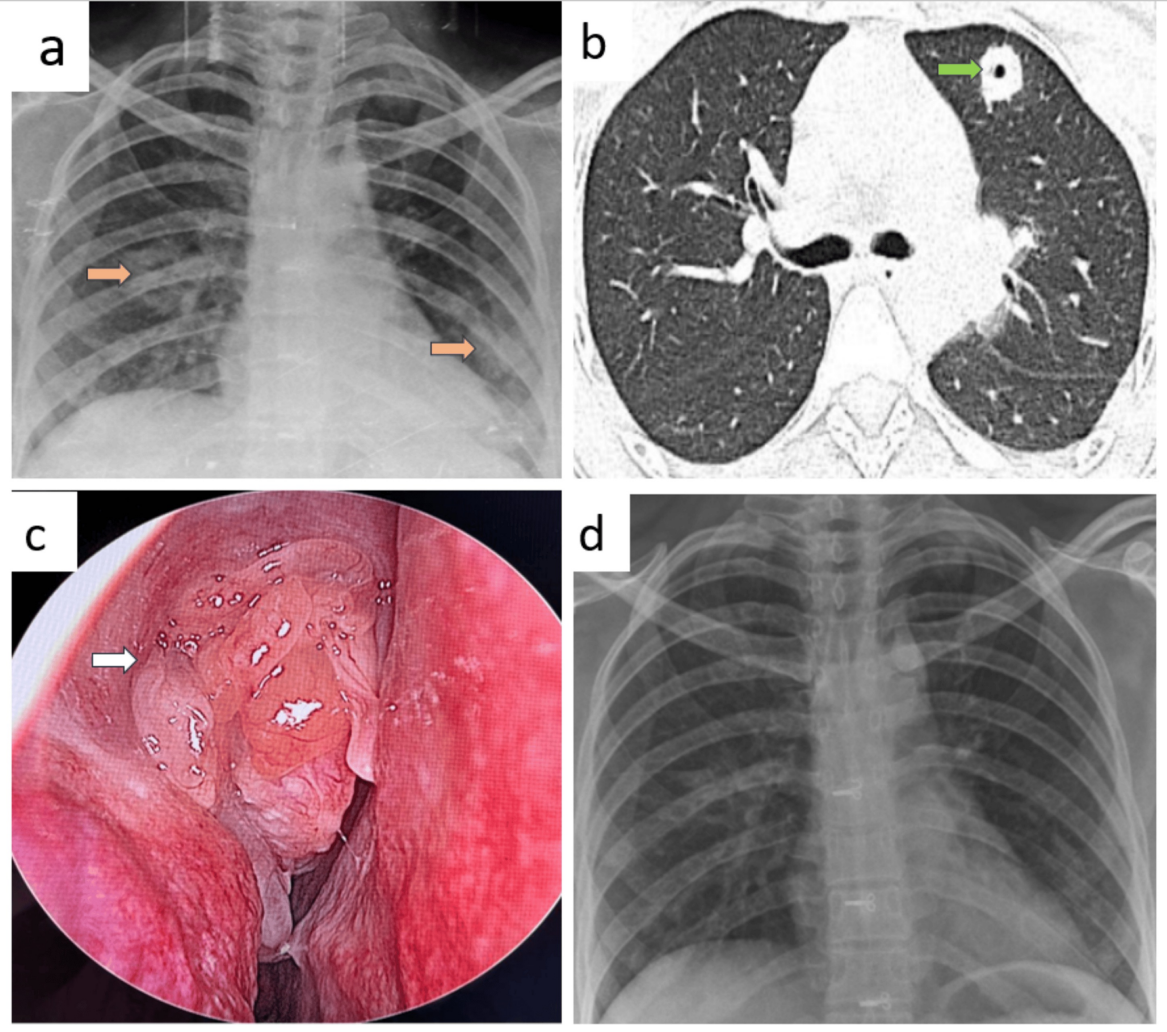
Radiology and anterior rhinoscopy images of patient C before and after treatment. (a) Chest X-ray imaging showed rounded non-homogenous opacities in the right upper and left lower lung zones marked by brown arrows. (b) CT thorax showed left upper cavitating nodule marked by a green arrow. (c) Anterior rhinoscopy examination showed right nasal polyposis marked by a white arrow. (d) Follow-up X-ray showed near complete resolution after four months.
